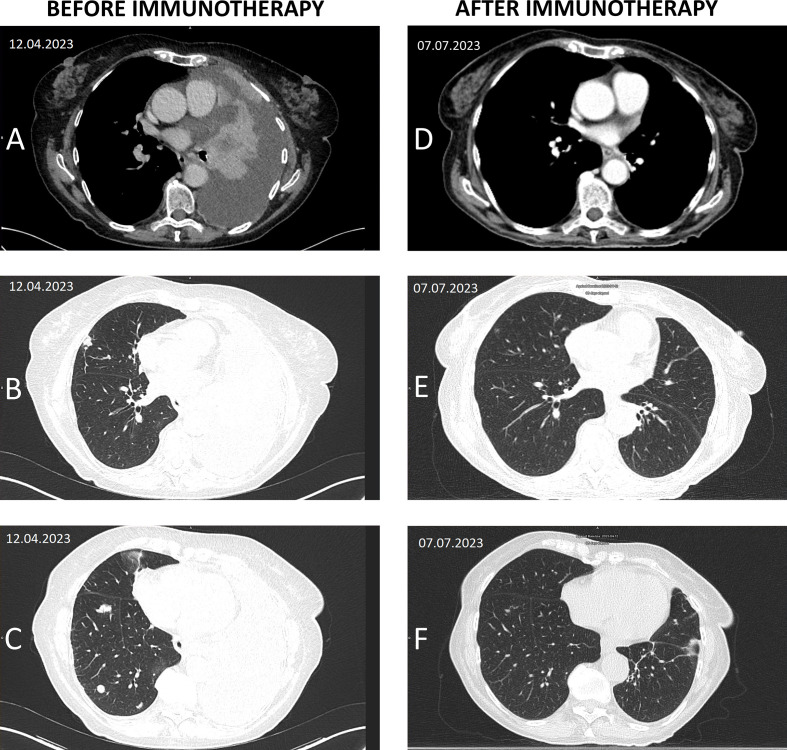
Thorax CT scan demonstrating severe left-sided pleural effusion [(A), soft tissue window] and multiple ATC lung metastases [(B, C), lung window]. Follow-up thorax CT examination performed three months after initiation of ICI treatment showing complete regression of metastatic lesions and pleural effusion (D–F).
